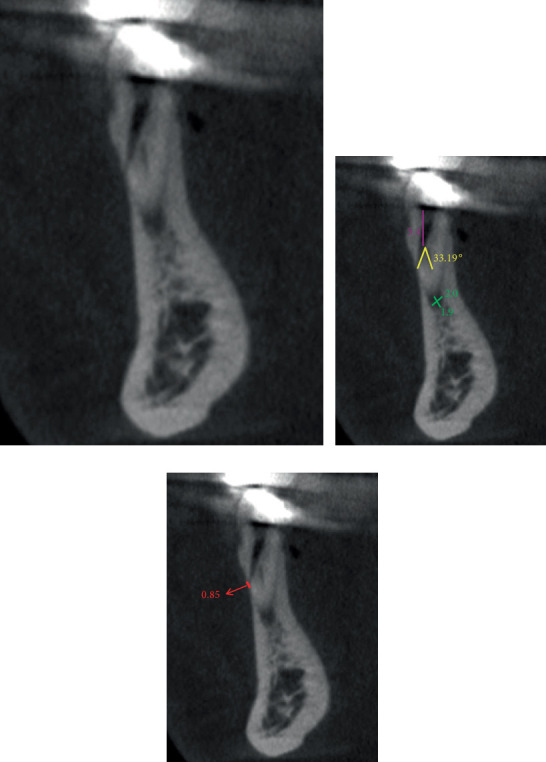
(a) CBCT image in sagittal section showing the mesiobuccal perforation in the tooth. An unprepared root canal was observed at the apex of Tooth Number 34. (b) In the sagittal section, the distance from the canal mouth to the perforation was 5.4 mm. The angle between the optimal entry vector and the unprepared root canal was 33.19°. Apical lesion measured 1.9 × 2.0 mm. (c) A lateral perforation of 0.85 mm in the apical distobuccal position.
